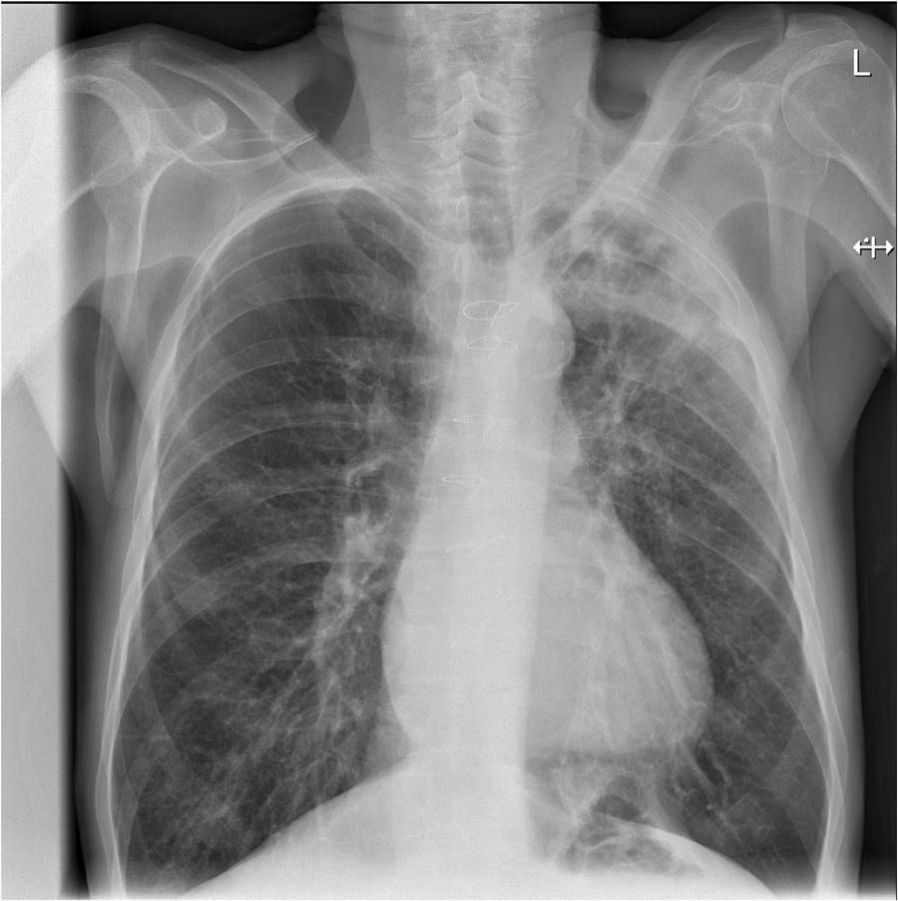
Posterior–Anterior chest radiograph showing a cavity at the left apex with surrounding consolidation. This was not present on the prior radiograph obtained nine months earlier.
